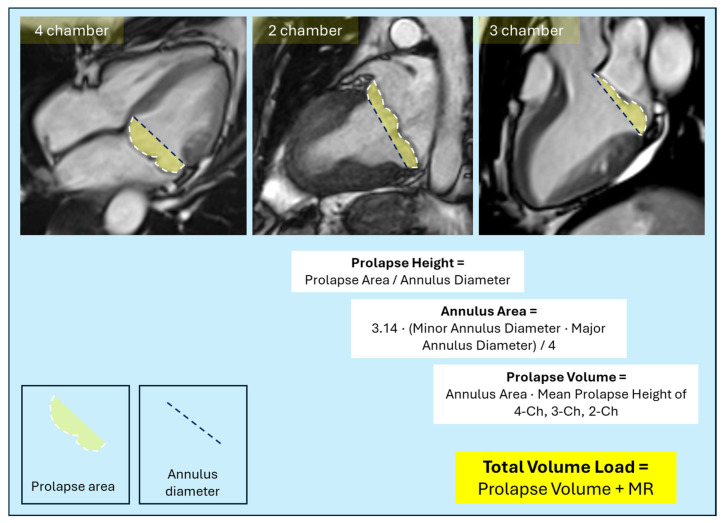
Cardiovascular magnetic resonance-based quantification of doming volume in mitral valve prolapse. Doming volume quantification using standard long-axis cine cardiovascular magnetic resonance images acquired at end-systole. Prolapse area is delineated in the four-chamber, two-chamber, and three-chamber views as the region bounded by the mitral annular plane and the ventricular surface of the prolapsing leaflets (green areas). The mitral annular plane is defined by a reference line connecting the medial and lateral annular hinge points. For each view, prolapse height is calculated as the ratio between prolapse area and annular diameter. Mean prolapse height is obtained by averaging measurements from the four-, three-, and two-chamber views. Mitral annular area is estimated assuming an elliptical geometry, using the three-chamber annular diameter as the minor axis and the larger of the four- or two-chamber diameters as the major axis. Doming volume is calculated as the product of mean prolapse height and annular area. The total volume load is defined as the sum of the doming volume and the mitral regurgitant volume.
